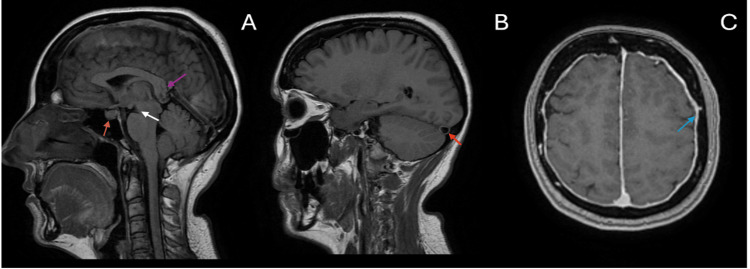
Sagittal T1 FLAIR images (A, B) demonstrating signs of intracranial hypotension, including drooping of the corpus callosum (pink arrow), midbrain sagging with reduced mamillopontine distance (3.5 mm) (white arrow), a bulky pituitary gland (orange arrow), and an engorged venous sinus (red arrow). Axial T1 post-contrast sequence (C) showing diffuse dural thickening and enhancement (blue arrow). FLAIR: Fluid-attenuated inversion recovery.
